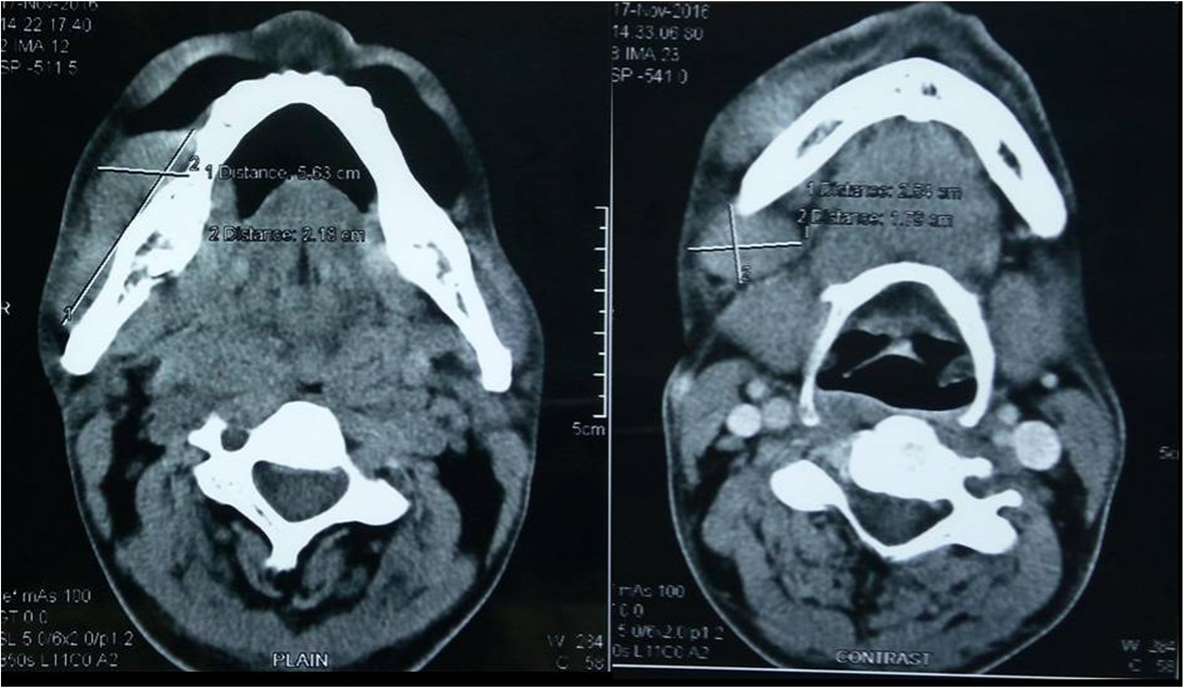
CT scan of the face and neck (Left) showing the lesion in the right buccal mucosa with extension onto the floor of the mouth and destruction of mandible (Right) showing the node in level Ib neck
